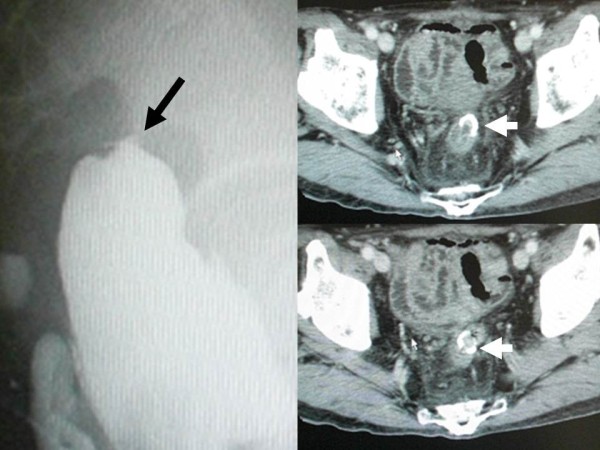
Findings of the fluoroscopy and abdominal computed tomography 6 months after the redo surgery. Fluoroscopy showed that anastomosis of the redo rectal resection was completely occluded (left, black arrow). Computed tomography showed that well-enhanced tissue was present inside the anastomotic circular stapler ring (upper and lower right, white arrows), suggesting that patency of the anastomosis was discontinued by the thick membranous web.
